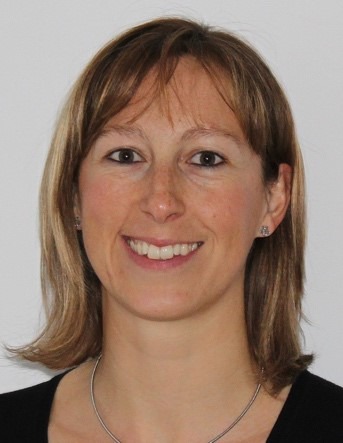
Dr. Nele Herregods obtained her MD at Ghent University in 2004 at the University of Ghent, Belgium. She received a PhD in 2016 at the same institution, with her doctoral thesis on MRI of the sacroiliac joints in children with spondyloarthritis, of which a summary is published in the current issue of the *JBSR* as a pictorial review article under the title “Diagnostic value of MRI of the sacroiliac joints in juvenile spondyloarthritis” [2]. She is currently working as head of clinic in the Department of Radiology at the Princess Elisabeth Children’s Hospital at Ghent University Hospital.
